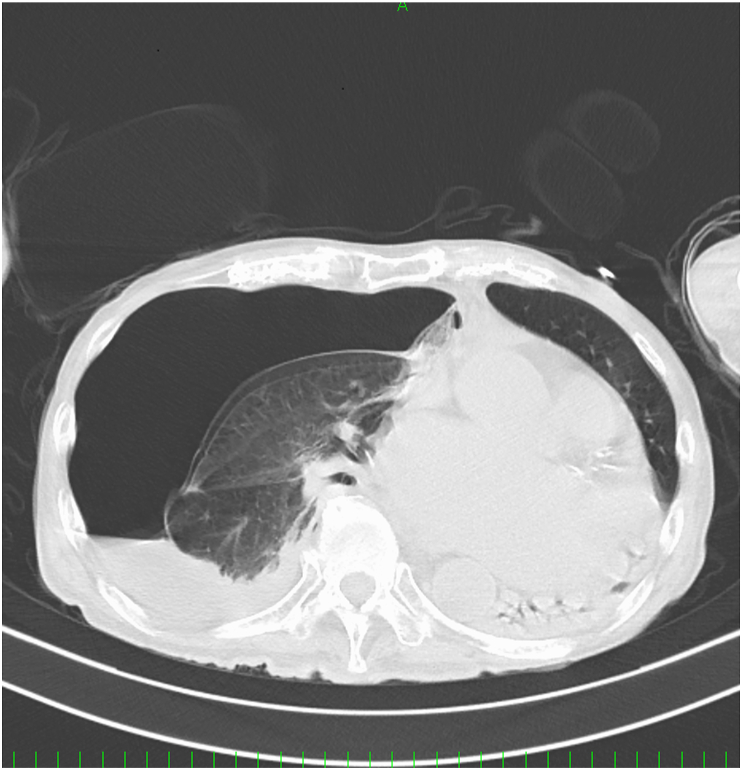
Computed tomography of pneumothorax in coronavirus disease 2019 (patient 7).
